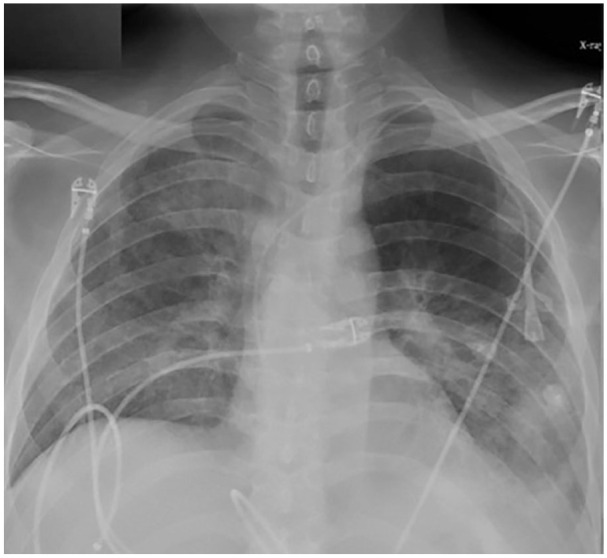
Initial chest X-ray obtained 35 minutes after bronchoscopy with transbronchial biopsy in the semi-upright position.
